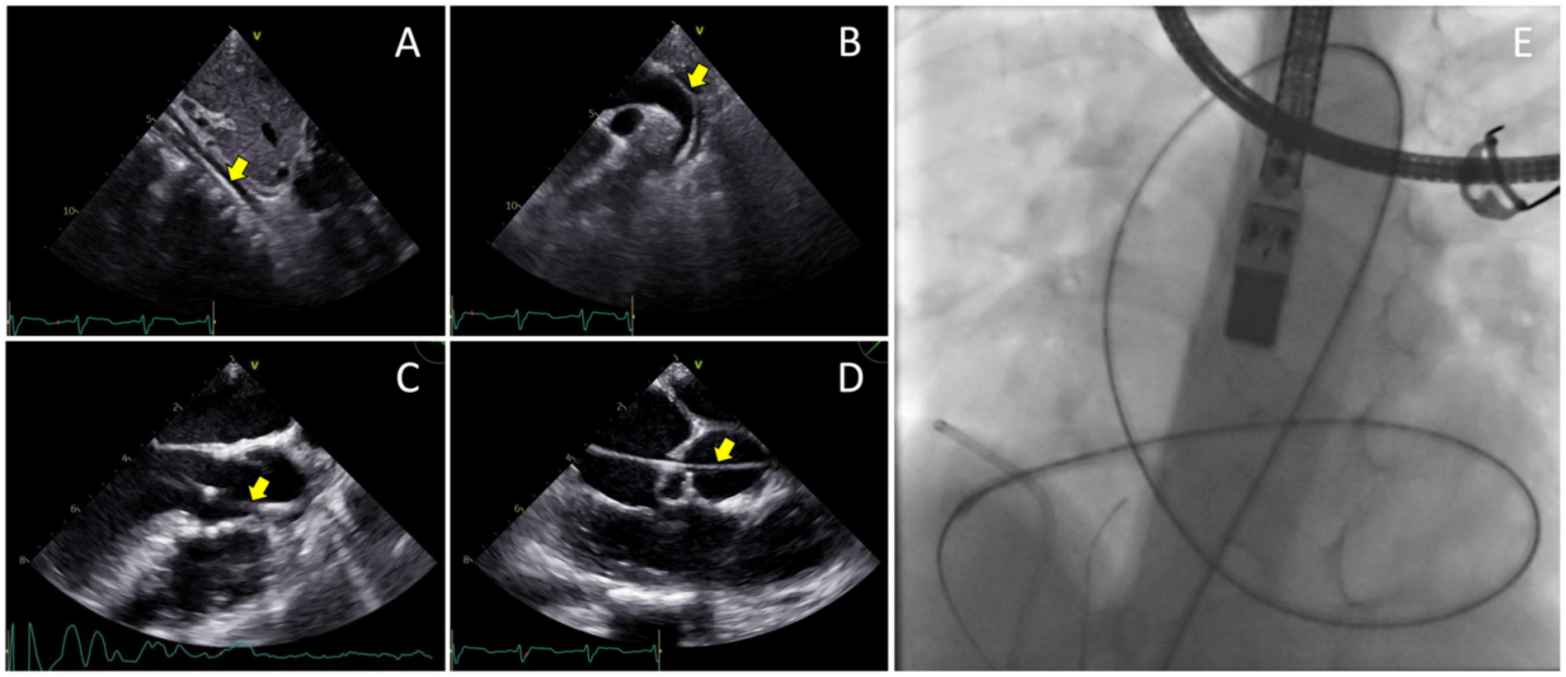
Transesophageal echocardiography views of the 0.035” soft hydrophilic wire (yellow arrows) being advanced from the femoral artery (A) into the abdominal aorta, (B) through the descending aorta and aortic arch, (C) into the left ventricle, (D) and crossing the tricuspid valve defect. (E) Fluoroscopy view of the Terumo wire after crossing the site of defect.
